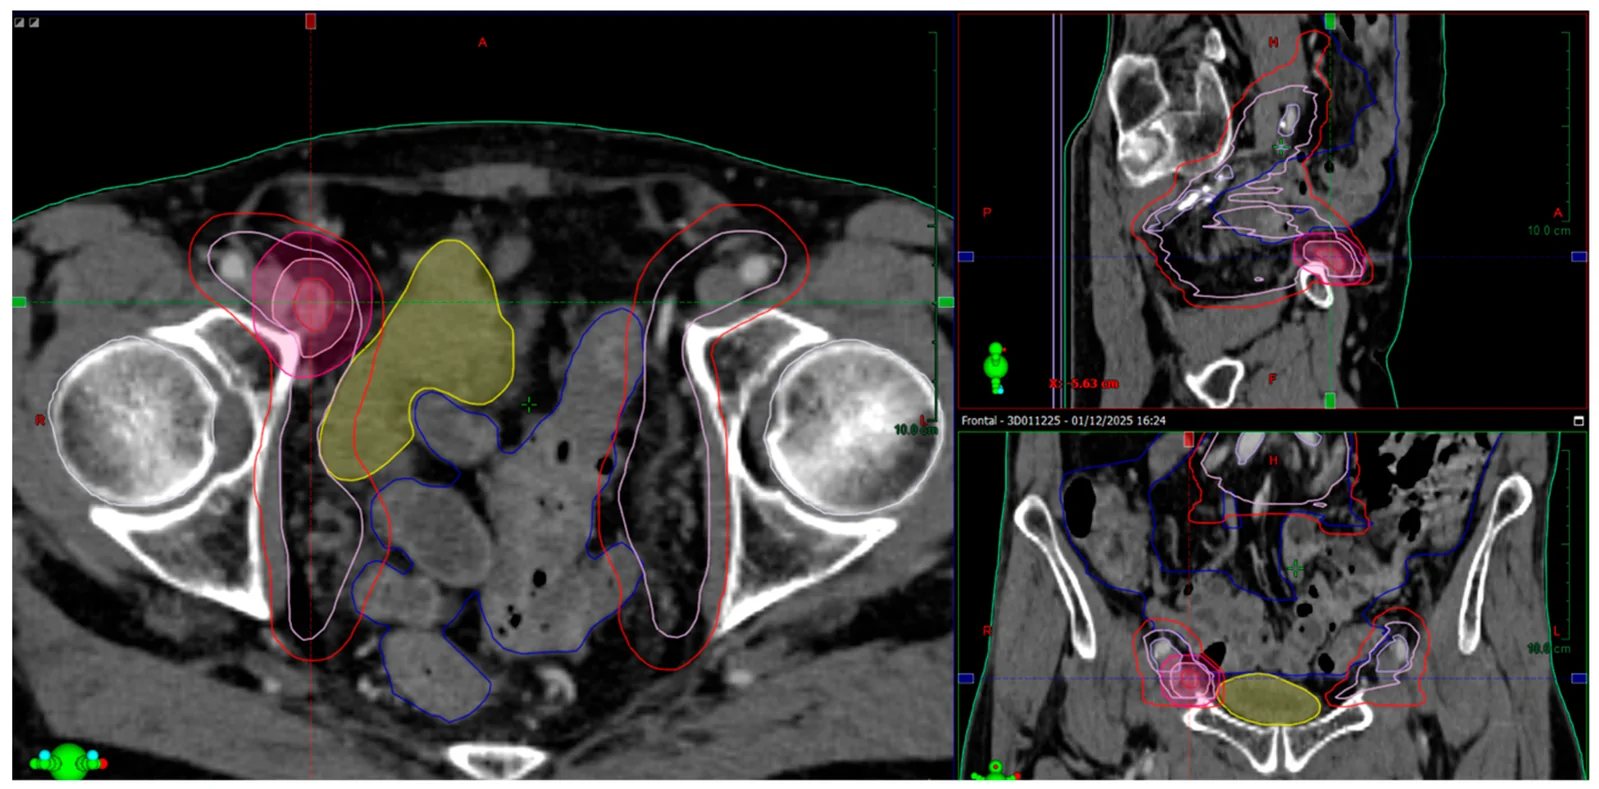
Pelvic radiotherapy with dose escalation to a PSMA-positive pathological lymph node.
